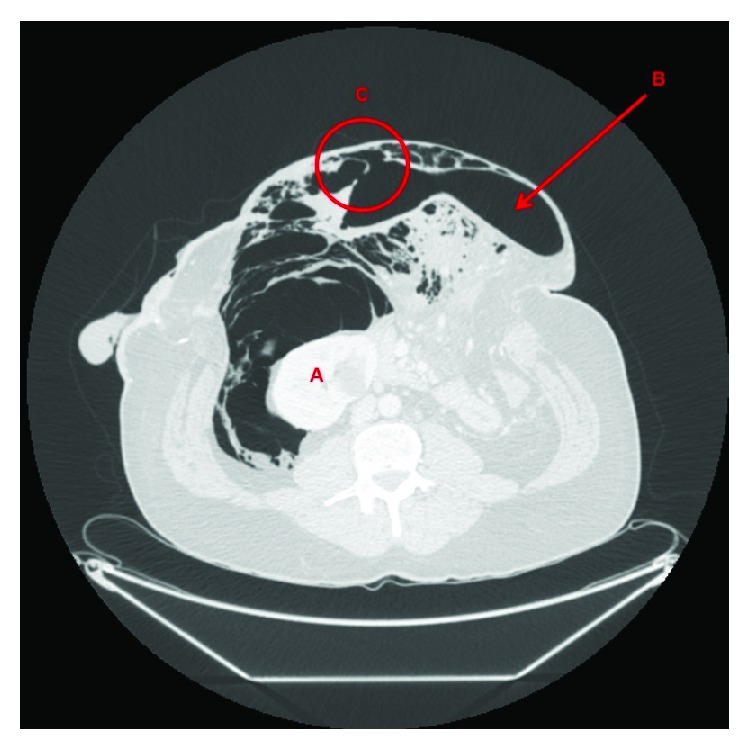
Axial CT image showing (A) retroperitoneal free gas around the right kidney (not typical with transverse colon perforation), (B) transverse colon, and (C) perforation of the transverse colon.
